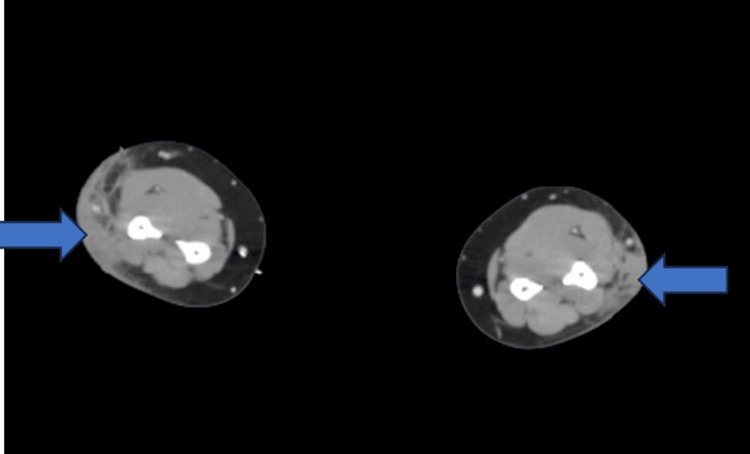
CT forearm showing subcutaneous nodules in the bilateral forearms with infiltrative changes (arrows showing the infiltrative changes)
